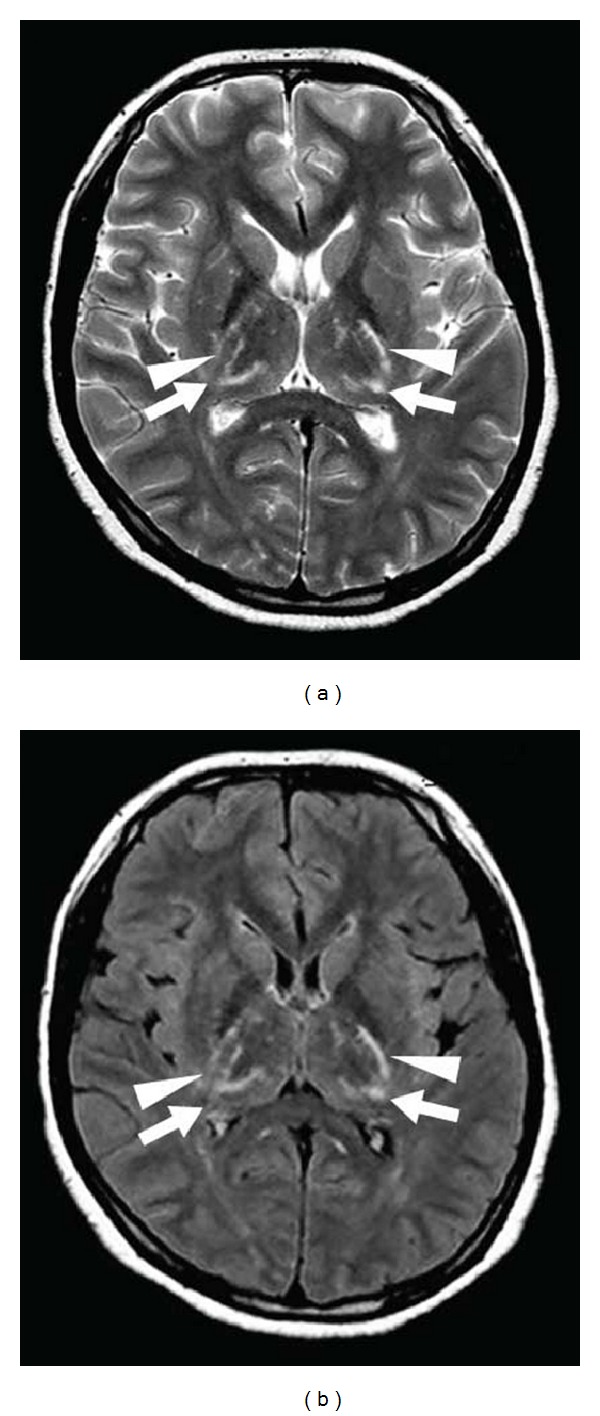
A patient with Mycoplasma pneumoniae-related acute necrotizing encephalitis involving symmetrically the posterior part of the internal capsule (arrowheads) and the posterolateral portion of the thalamus (arrows) on both sides, seen as hyperintensity on both T2-weighted (a) and FLAIR (b) imaging.
